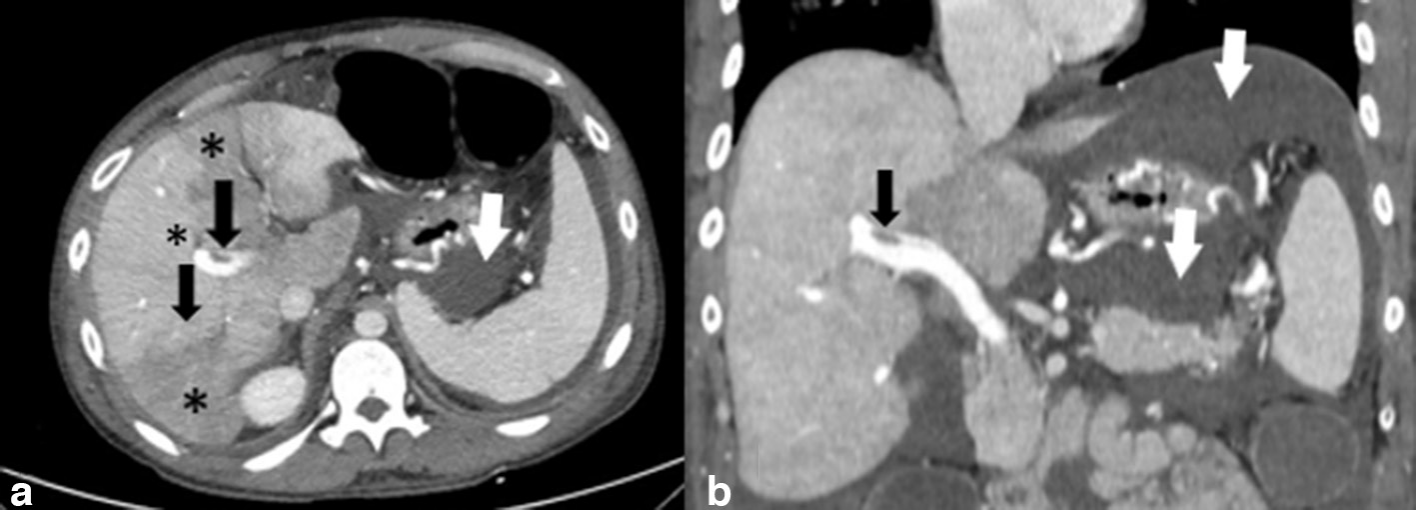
Axial (a) and coronal (c) contrast-enhanced CT images of the abdomen demonstrate a clot within the portal vein (black arrows). There are altered patchy areas of hepatic parenchymal enhancement (*) that are suggestive of occlusion of small branches of the portal vein. Intraperitoneal fluid is also seen on CT images (white arrows).
